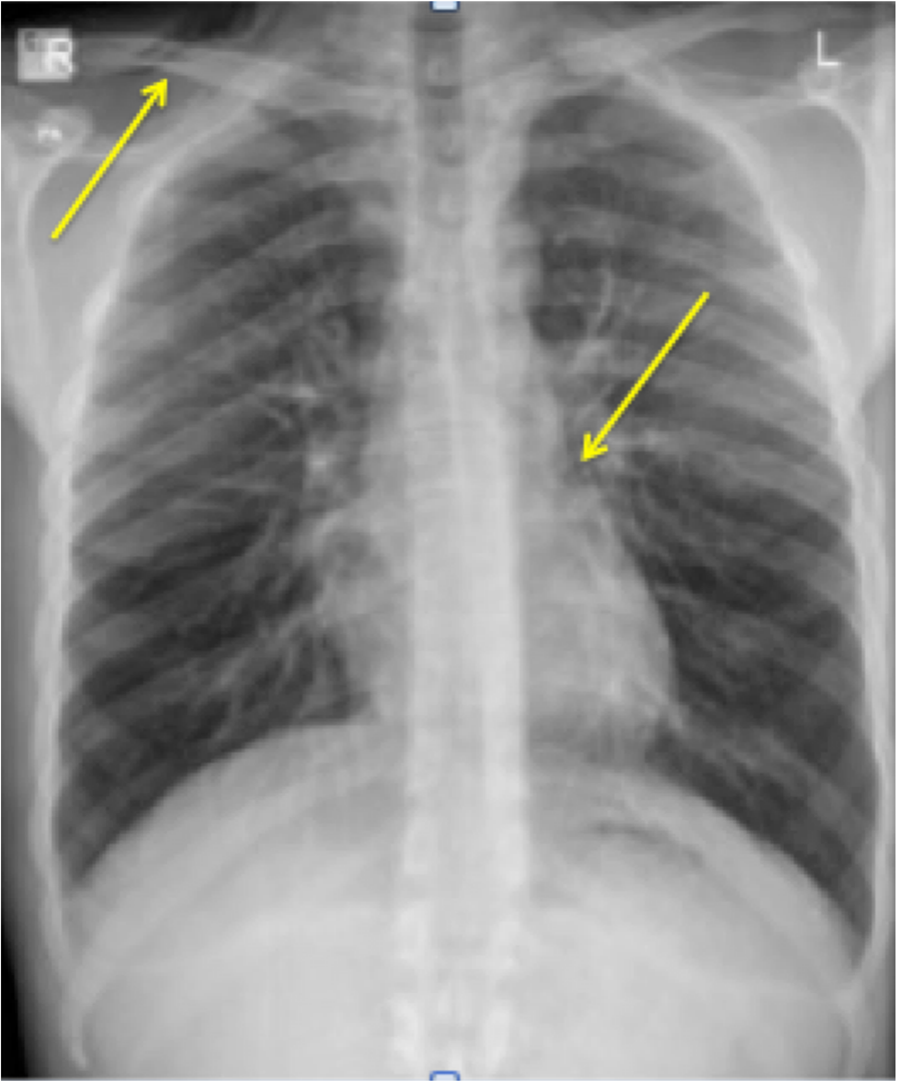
Plain posterioranterior chest radiograph showing pneumomediastinum and subcutaneous emphysema (bottom arrow and top arrow respectively)
